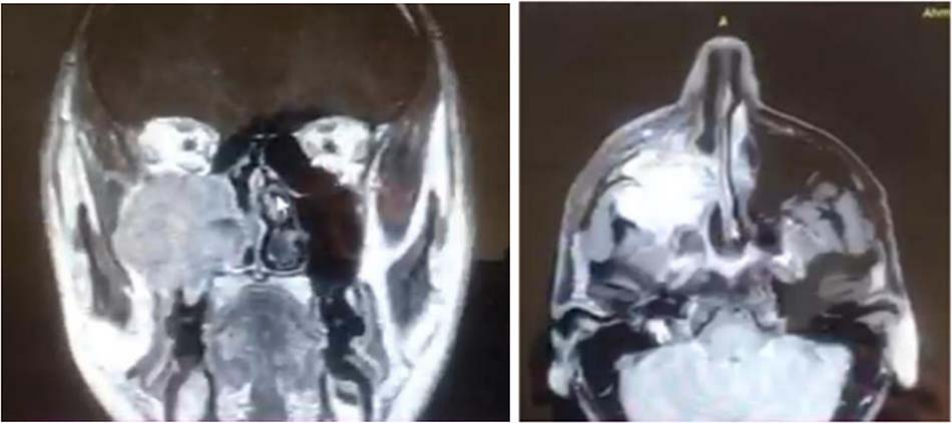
MRI image of right maxillary sinus-centered AC.
